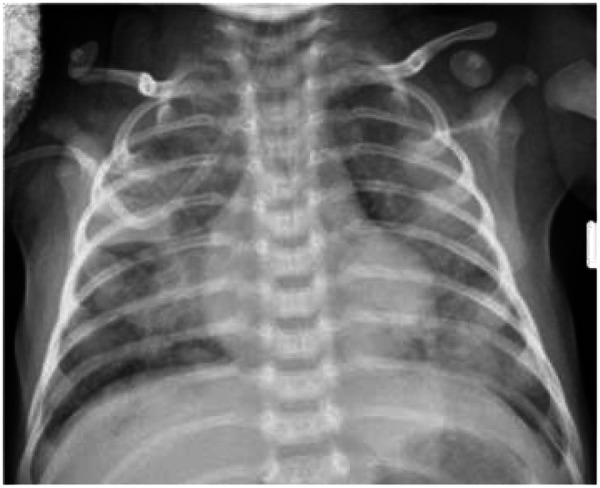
Chest x-ray reveals multiple patchy opacities in both lungs.
